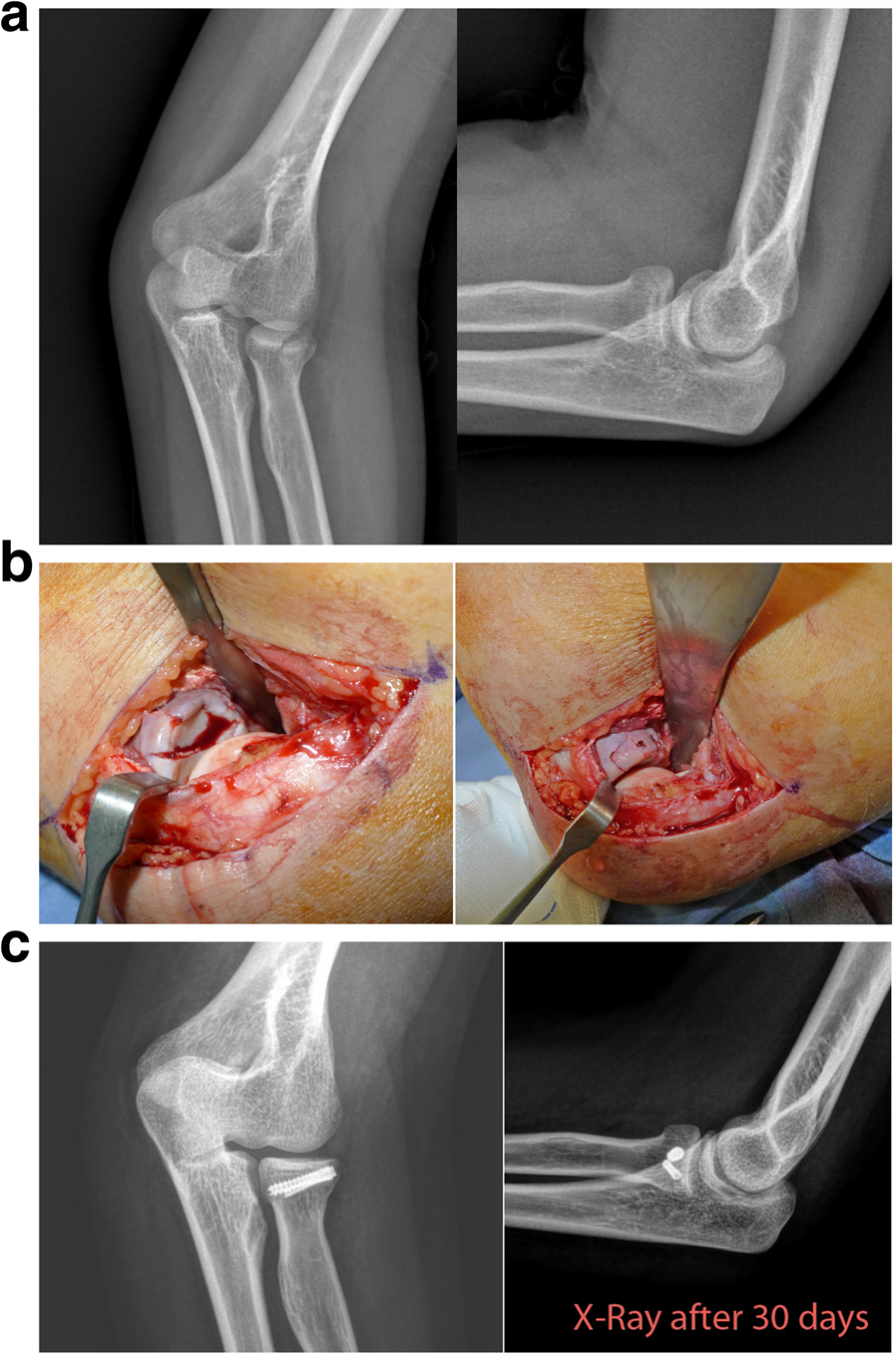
a X-rays showing a Mason type II fracture. b Intra-operative view showing the reduction and fixation using 2 mini-screws. c X-rays performed after 30-days with signs of fracture healing and reduction maintenance
